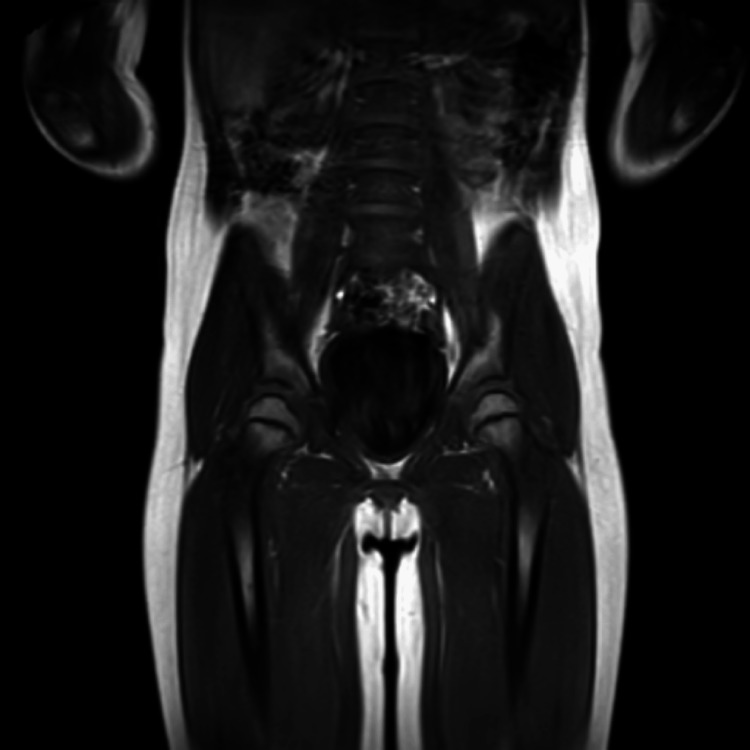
MRI study (T1) after five cycles of tocilizumab desensitization, with the disease in a non-active state. The lower dorsal region, lumbar spine, sacrum, and coccyx show no abnormalities in vertebral bodies or intervertebral spaces. The spinal cord and cauda equina appear normal. The sacroiliac and hip joints show no alterations and no evidence of edema or joint effusion. The thigh muscles up to the mid-third of the femur show no abnormalities, and the signal intensity of the femur up to the mid-third is normal.
